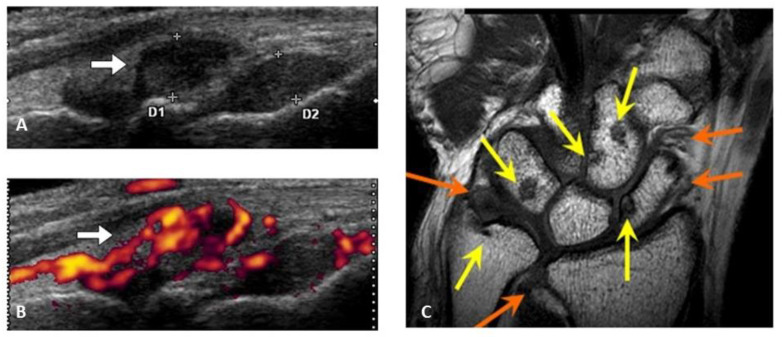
Rheumatoid synovium by ultrasonography and bone erosion by MR-imaging. Synovial hypertrophy (white bar) by systematic multiplanar grayscale ultrasonography (A), marked signals of synovial flow by power doppler ultrasonography (B), bone erosion ((C), yellow bar) and synovial hypertrophy ((C), orange bar) by MR-imaging are characteristic images in rheumatoid arthritis.
